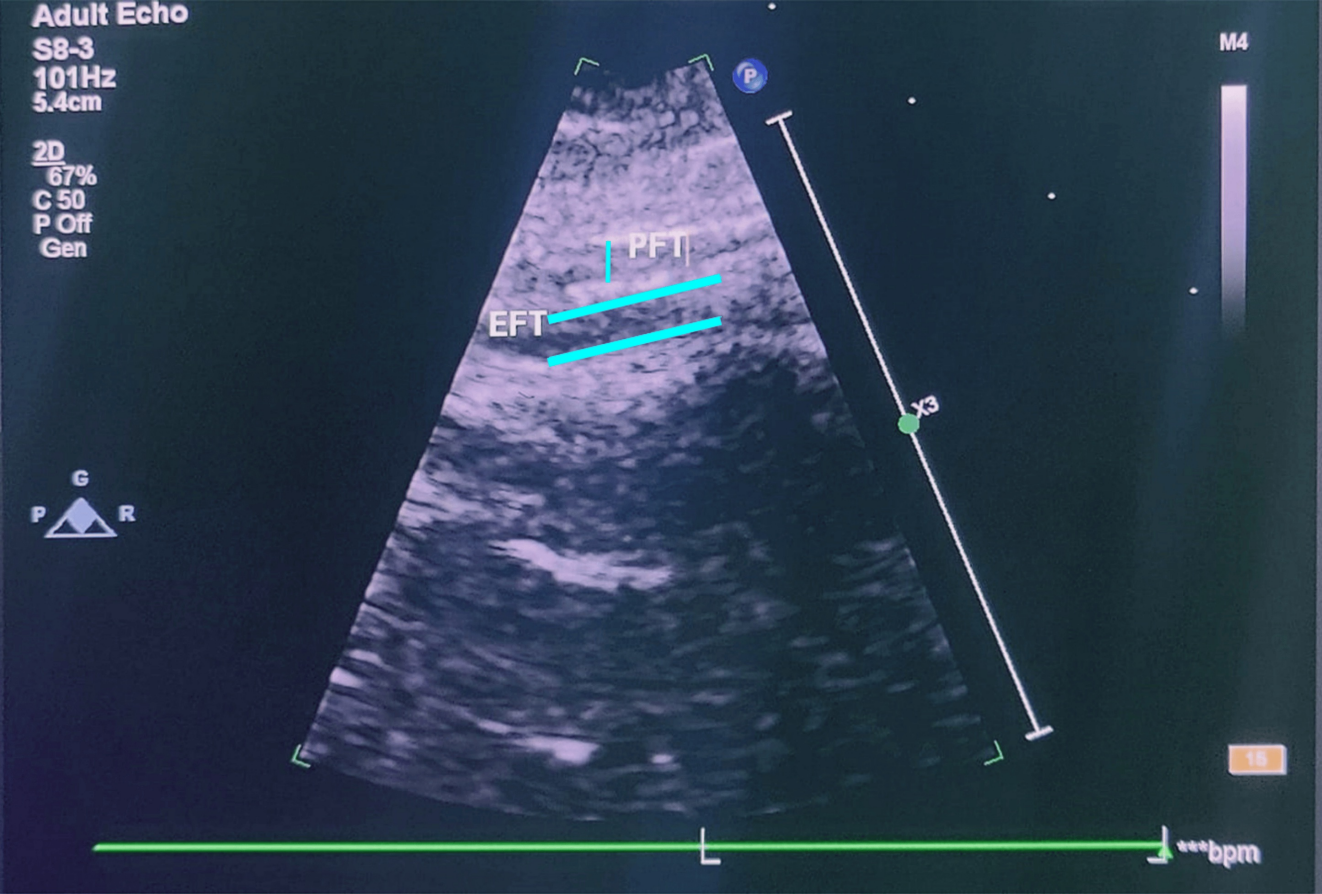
Epicardial fat thickness assessment on echocardiography. EFT: epicardial fat thickness; PFT: pericardial fat thickness.
